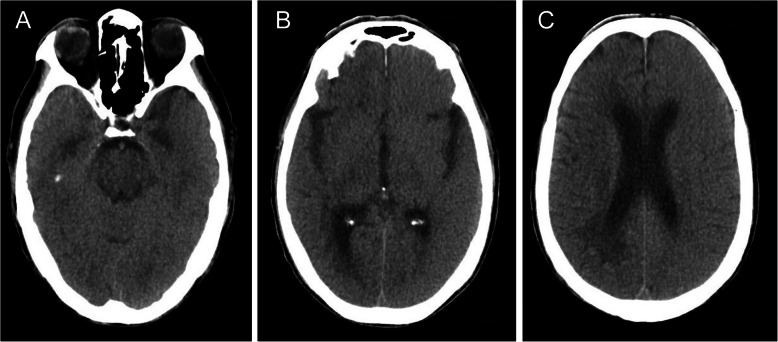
CT scan of the patient’s head after treatment. a-c: The high-density shadow disappeared, and the oedema was significantly absorbed
